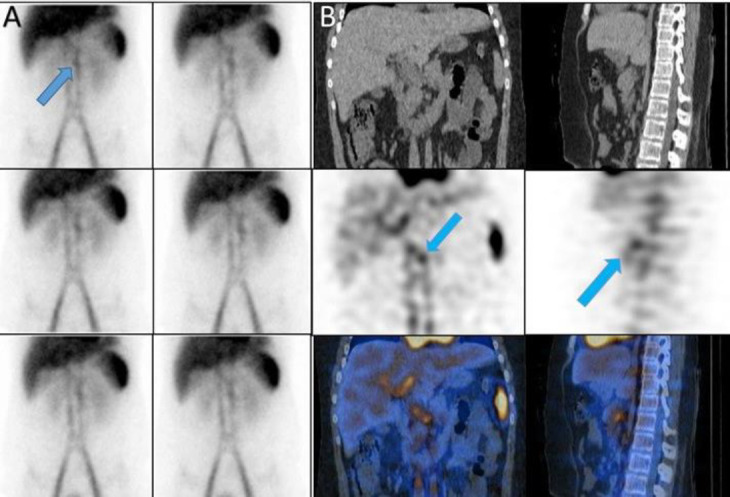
Celiac artery on planar (A) and SPECT/CT (B)
